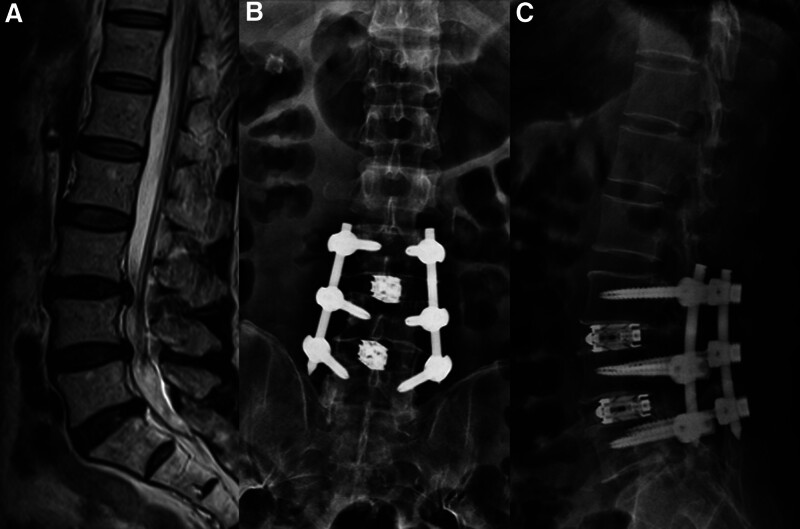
(A) Preoperative MR; (B) and (C) postoperative X-ray. MR = magnetic resonance.
